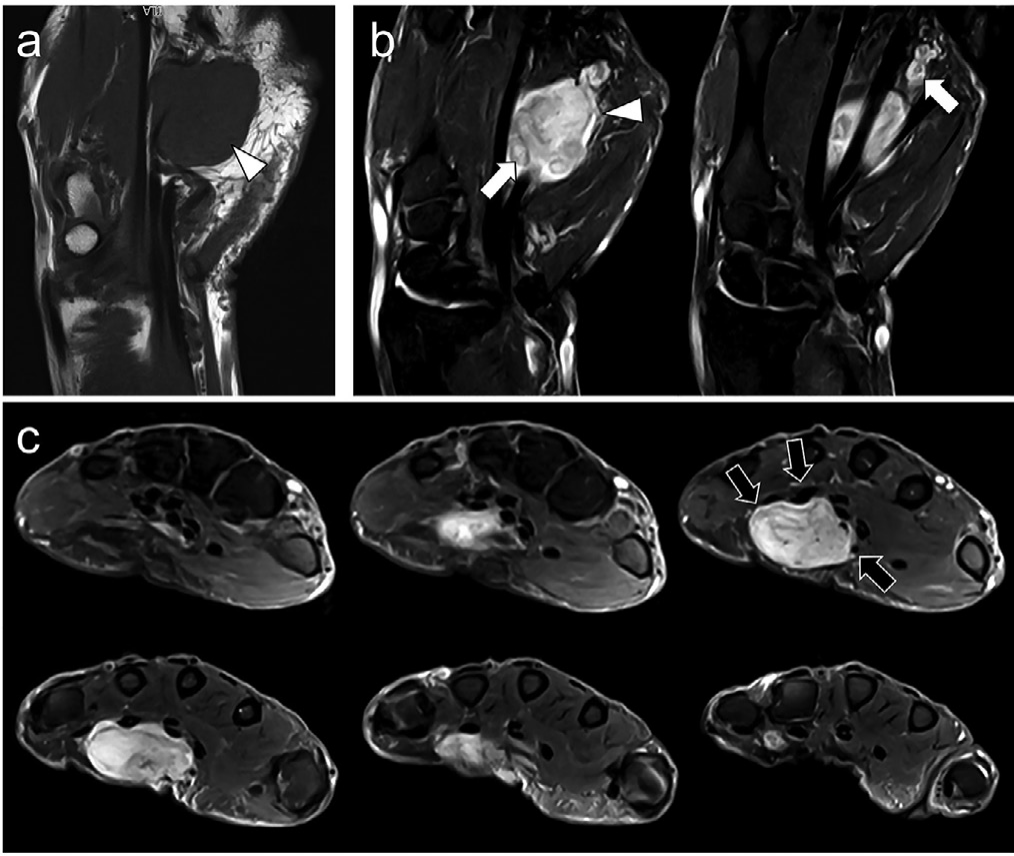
A 72-year-old male with a swelling of the ulnar palmar side of the hand underwent a conventional MRI. (a) Coronal *T*_1_WI showed a 54 mm-long, well-defined, non-encapsulated single tumor with SI similar to normal muscle, without fat or hemorrhage (white arrowhead). (b) Coronal fat-suppressed *T*_2_WI demonstrated high SI and intratumoral nodule delineated by thin strand of lower SI on *T*_2_WIWI (white arrows) leading to a slight heterogeneity. (c) On consecutive axial fat-suppressed *T*_2_WIWI, the tumor demonstrated a close relationship to superficial digital flexor tendons as well as superficial palmar arch with extensions following the third, fourth and fifth fingers. There was no surrounding edema or bone invasion. SI, signal intensity; *T*_1_WI, *T*_1 _weighted imaging;*T*_2_WI, *T*_2 _weighted imaging.
